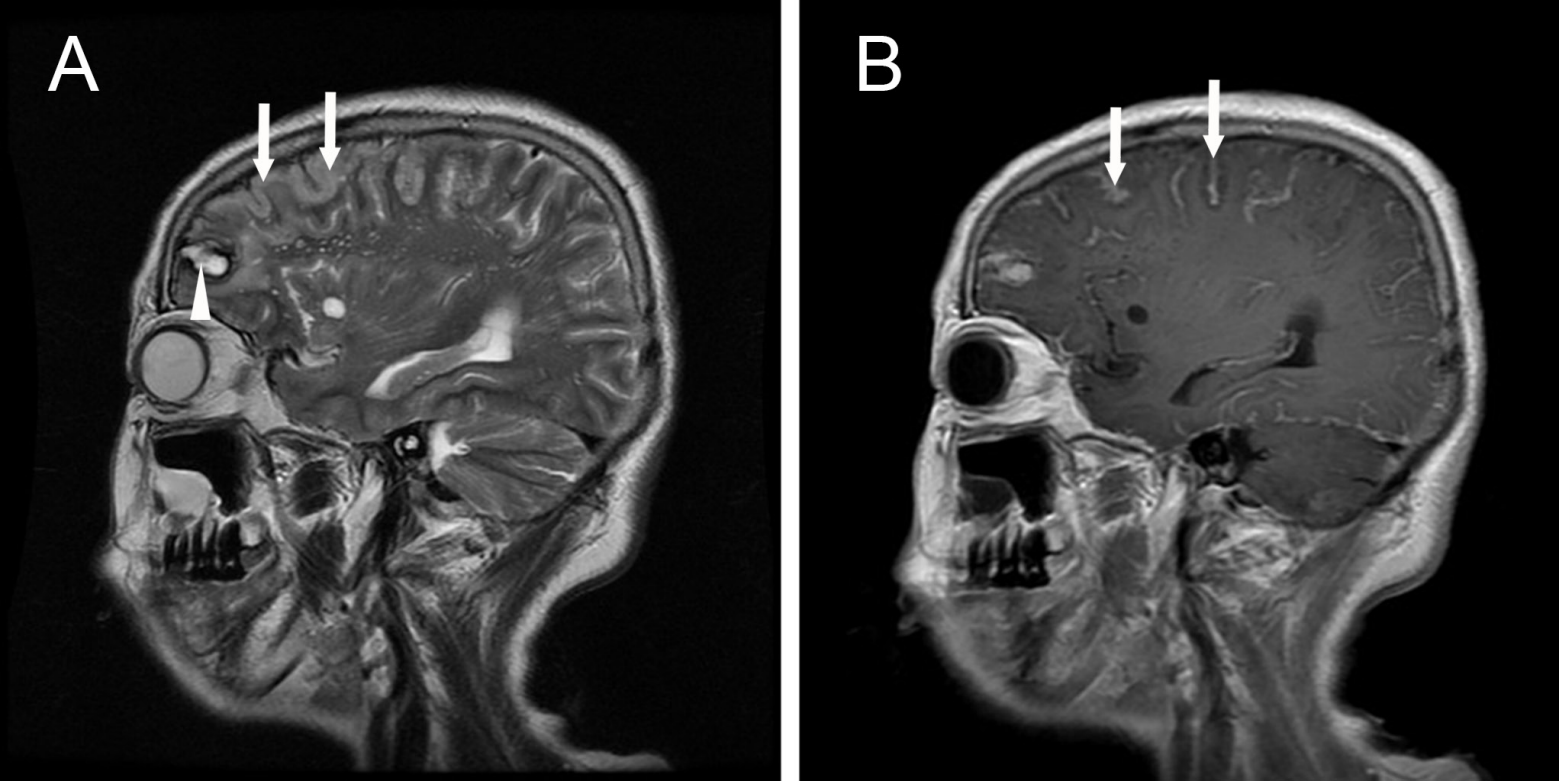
Sagittal brain magnetic resonance images A. T2-weighted magnetic resonance imaging shows a hematoma (arrow head) in the frontal lobe and cerebral gyrus swelling (arrows); B. T1-weighted magnetic resonance imaging with contrast shows abnormal leptomeningeal enhancement (arrows).
